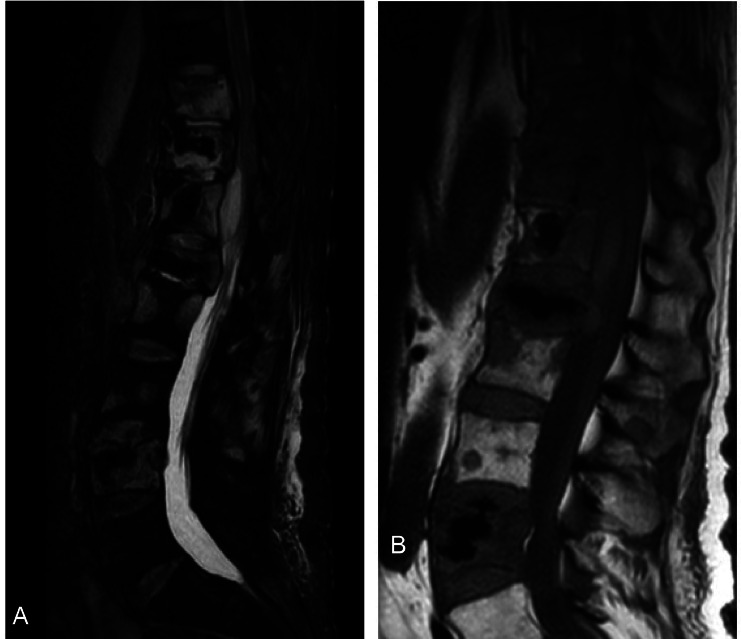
Sagittal T2 (A) and T1 (B) contrast enhanced MRI of the thoracolumbar spine showing similar enhancement between the infected vertebrae (T9 and T10) and the metastatic lesions distal to that.
